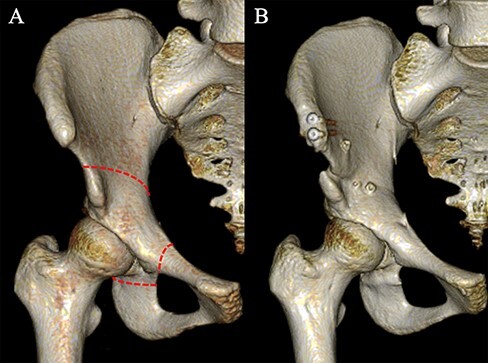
Preoperative and postoperative three-dimensional CT of the pelvis in the AP view. (A) Preoperative pelvis. The graphic shows the osteotomy line of CPO (dotted line). (B) Postoperative pelvis. The acetabular fragment was rotated laterally (to make the weight-bearing area horizontal) and fixed with three poly-l-lactic acid screws.
